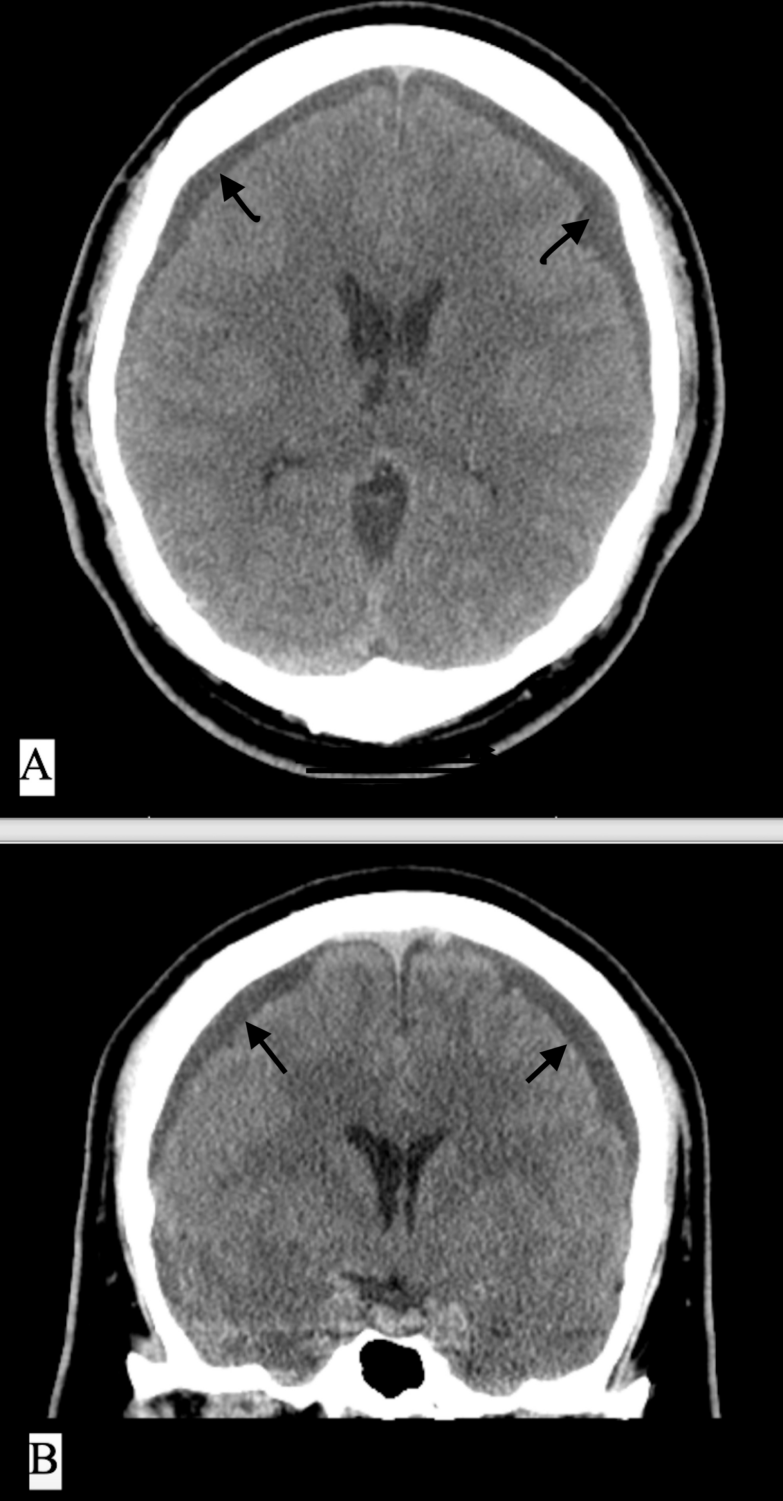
Initial CT imaging during headache workup; evidence of chronic bilateral subdural fluid collections A) CT of the head, axial cut, non-contrast; B) CT of the head, coronal cut, non-contrast
